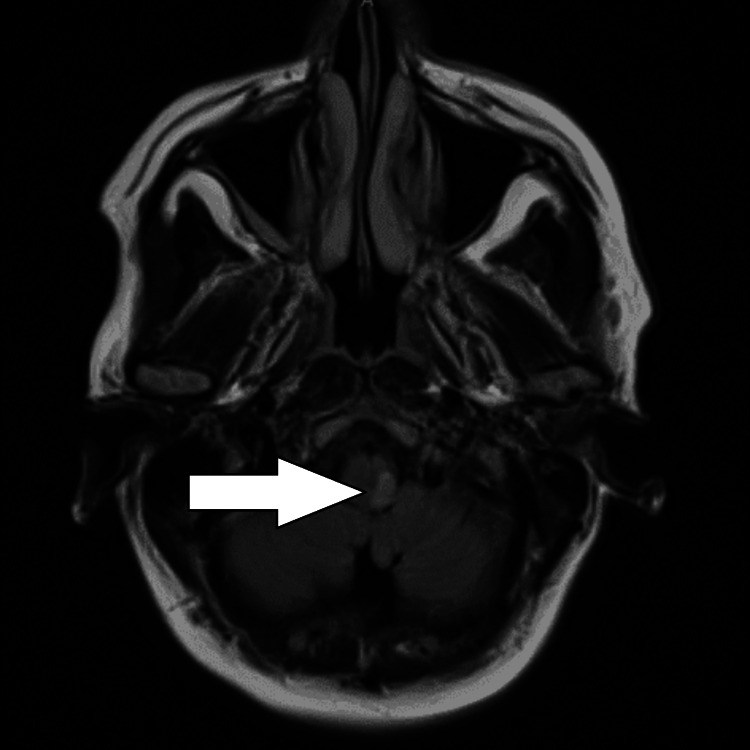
MRI T2 FLAIR The restricted area of diffusion in the medulla on the left side is also visible on the T2 FLAIR, which is consistent with an acute medullary infarct on the left side. The hyperintensity seen in the T2 FLAIR is known to be associated with stenosis and occlusion of vessels and the attendant flow. This is indicated by the arrow in the figure. MRI = Magnetic Resonance Imaging; FLAIR = Fluid-Attenuated Inversion Recovery
